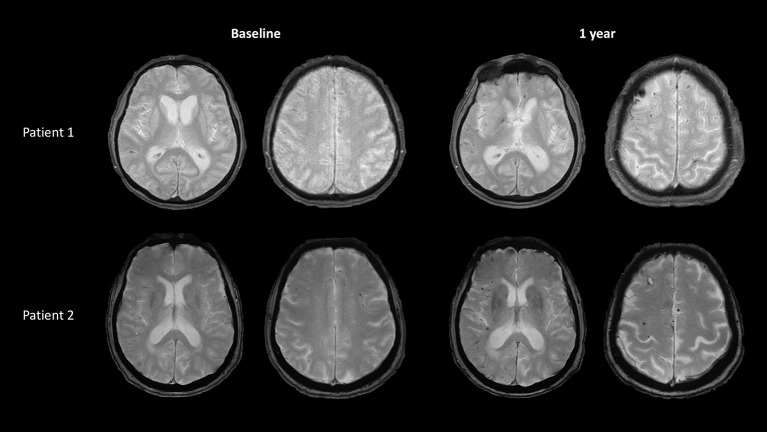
Follow-up brain MRI in the 2 patients. T2* sequences at 1 year after the onset, in patient 1 and patient 2 showing increasing load of superficial microbleeds and hemosiderosis.
